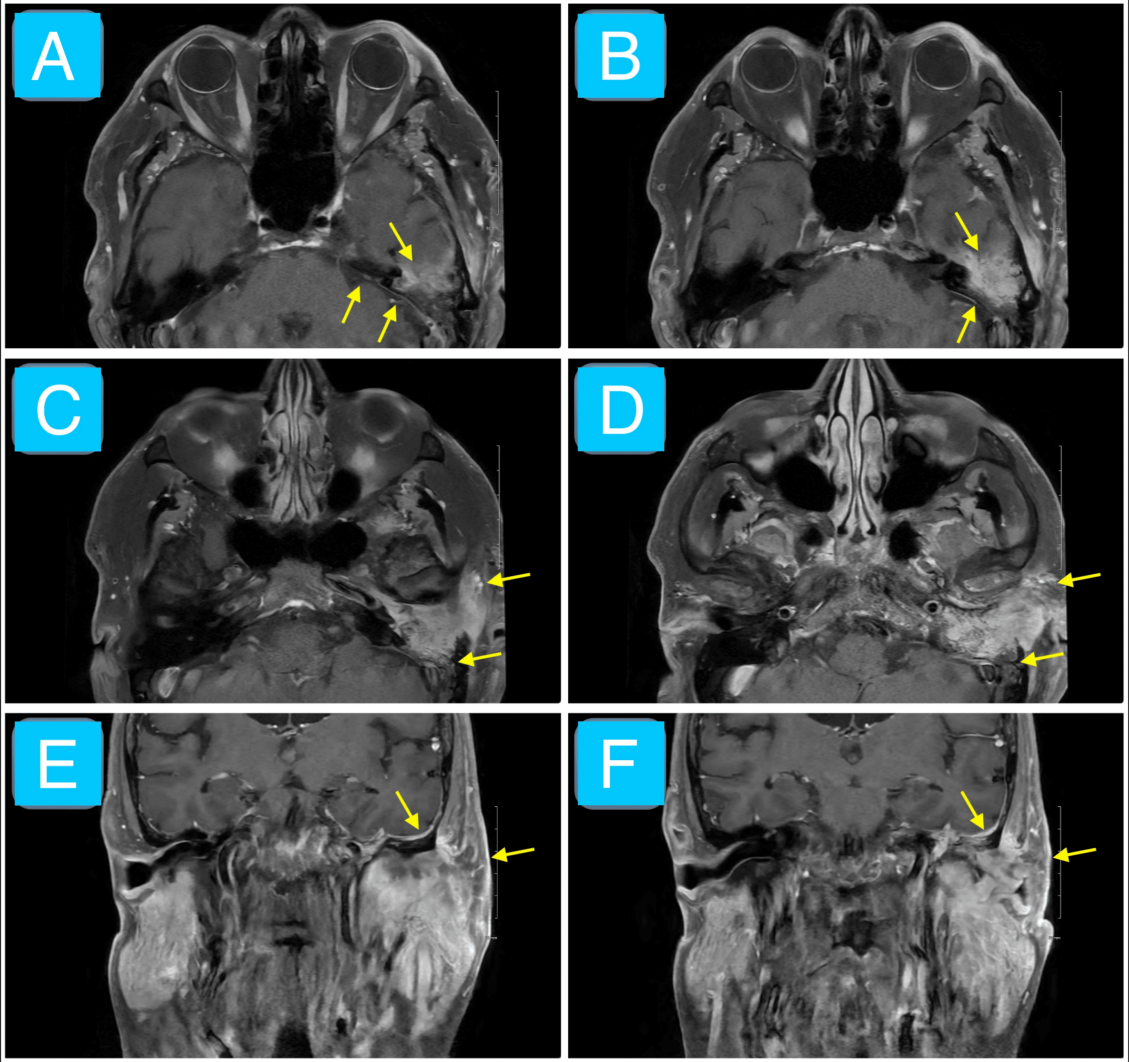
Contrast-enhanced T1-weighted MRI of the face Post-contrast axial (images A-D) and coronal (images E-F) T1-weighted sequence MRI of the face in a 63-year-old male showing a 3.6 × 1.8 × 3.0 cm continuously enhancing lesion invading the left EAC, middle ear, petrous apex, mastoid bone, posterior TMJ wall, temporal lobe (with local dural enhancement), facial nerve canal, and retromandibular space (yellow arrows). These findings are consistent with an aggressive mass. Biopsy confirmed moderately differentiated SCC. EAC: external auditory canal; TMJ: temporomandibular joint; SCC: squamous cell carcinoma
